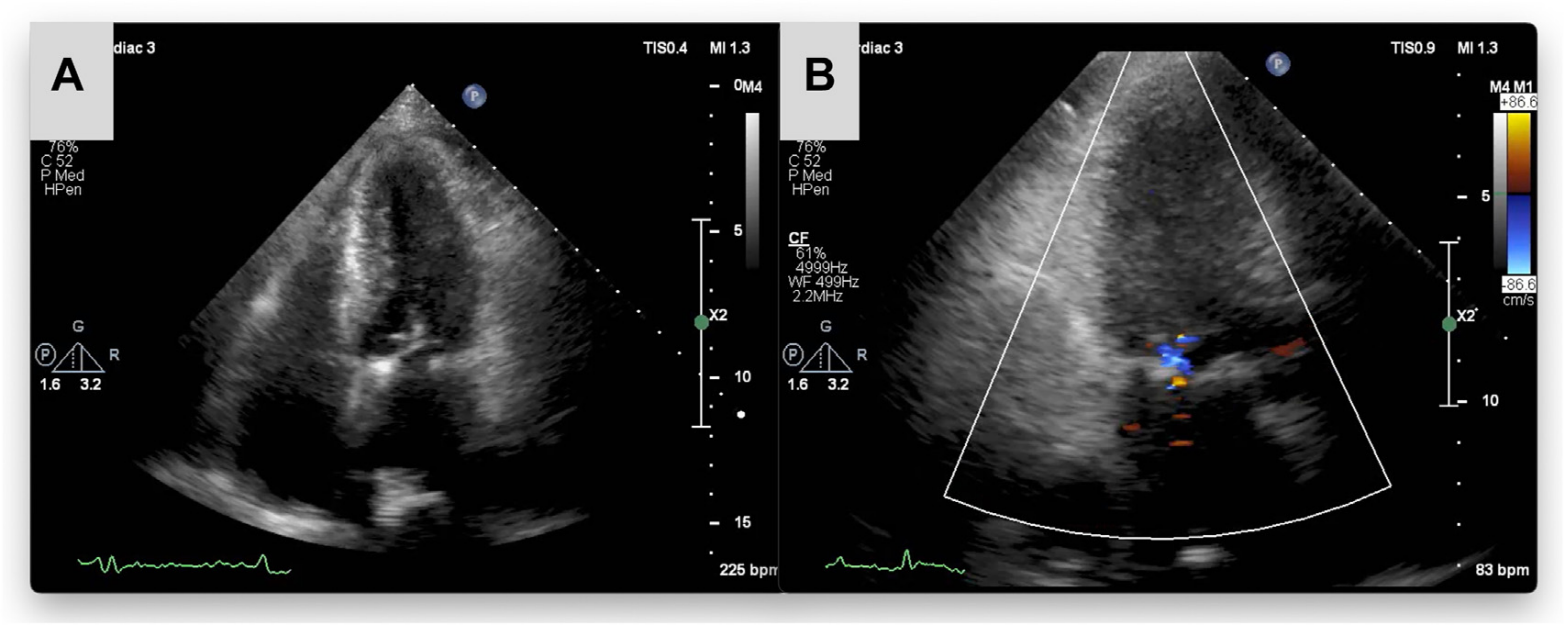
(A) Postoperative transthoracic echocardiography shows repair of the anterior leaflet prolapse. (B) Findings show that the mitral regurgitation is reduced to mild severity.
